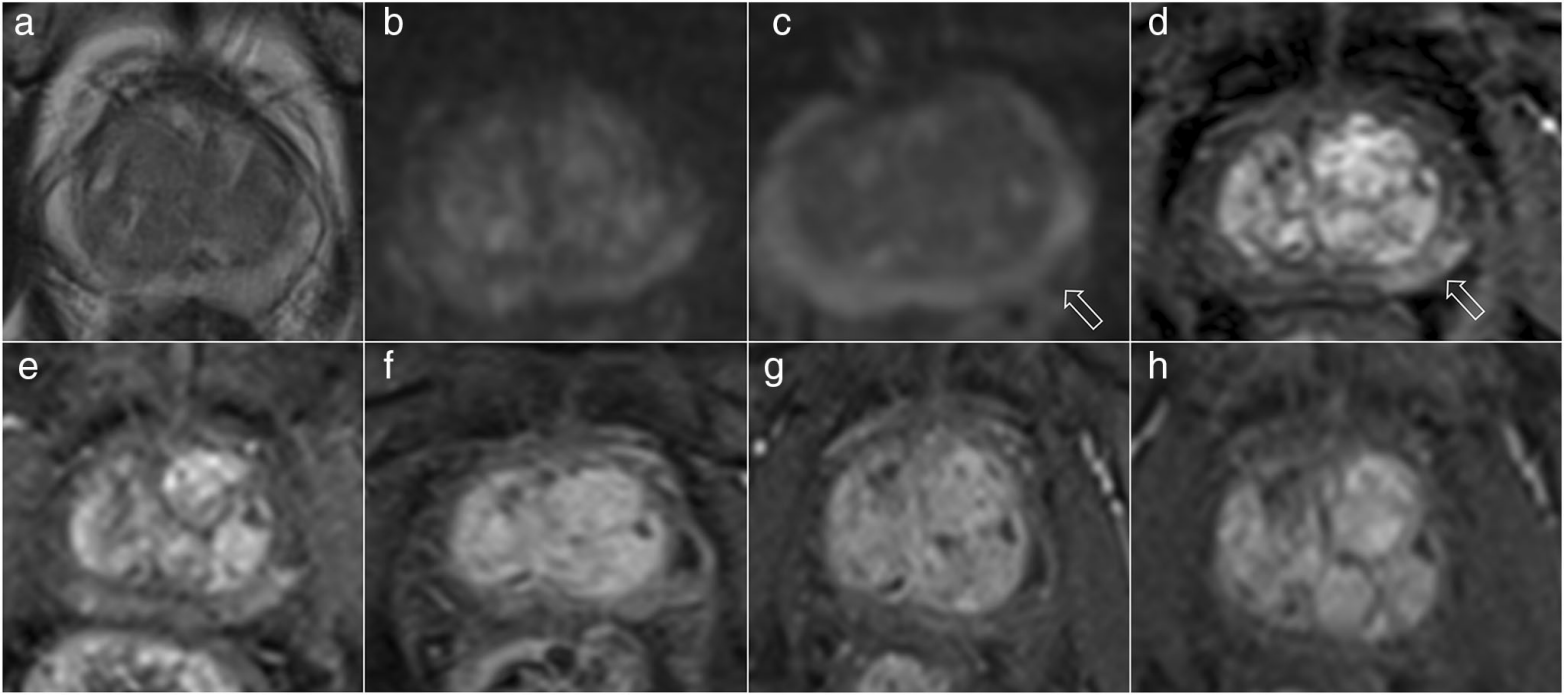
66-year-old patient on active surveillance for Gleason 3 + 3 (2 mm) in the left midgland peripheral zone on standard transrectal ultrasound biopsy and a presenting PSA of 13 ng/ml (PSA density, 0.17). The first 1.5-T MRI scan (a–d) shows a left-sided peripheral zone area (arrows) characterised by mild restricted diffusion on the ADC map (c) and early enhancement on dynamic contrast-enhanced imaging (d). The area showed stable MR appearance on dynamic contrast-enhanced imaging after one year (e) (scored as PRECISE 3 by both radiologists) and radiological regression (PRECISE 2) after one (f) and two years (g). The last scan (h) shows resolution of the previous suspicious MR features (PRECISE 1), with a PSA of 14 ng/ml and a PSA density of 0.16. The patient was discharged to his general practitioner for follow-up
